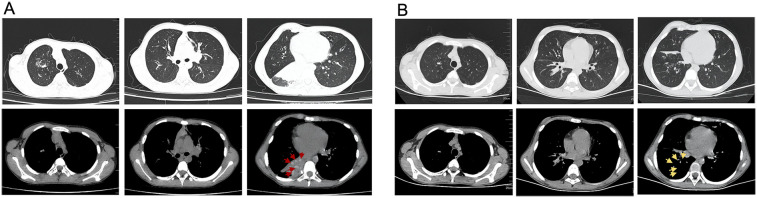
Changes on chest CT before and after treatment. (A) Chest CT scans showing right lower lobe atelectasis and high-density shadows in the bronchus of right lower lobe before treatment. The red arrows indicates the casts with a CT attenuation value of 81 HU in the mediastinal window. (B) Chest CT scans showing re-expansion of the right lower lobe after treatment. Post-clearance CT changes are demarcated by yellow arrows.
